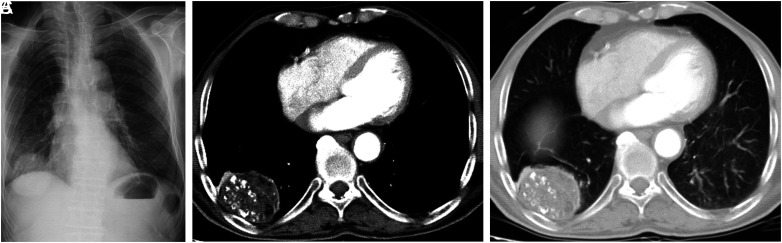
Chest x-ray radiography (a), chest CT scan in mediastinum window (b), and parenchymal window (c) sections show a solid mass with amorphous hyperdense calcified components in the right lower lobe superior segment in an 80-year-old male patient. CT, computed tomography.
